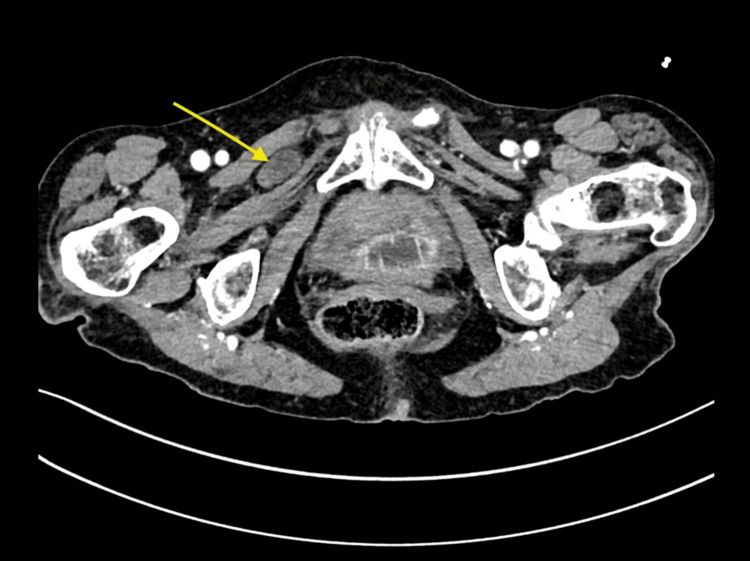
Axial CT scan showing right obturator hernia with incarcerated ileal loop This axial slice from a contrast-enhanced abdominal CT demonstrates a loop of ileum (arrow) herniating through the right obturator canal. The ileal loop is located between the pectineus and the obturator externus muscle. CT: computed tomography
